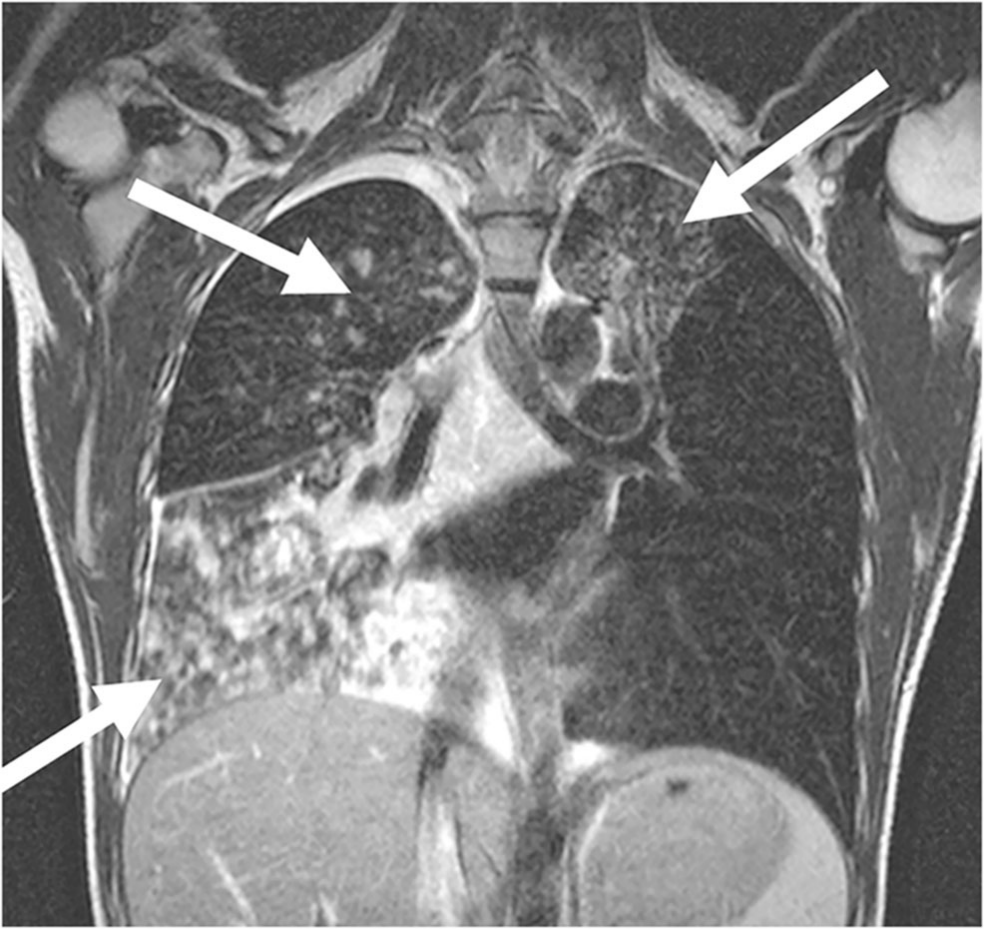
MRI of tuberculosis in a 12-year-old girl. Coronal T2-weighted turbo spin-echo image (MR parameters in Table 2) demonstrates that in older children, the tuberculous infiltrates (*arrows*) are often multi-focal and look similar to bronchopneumonia
